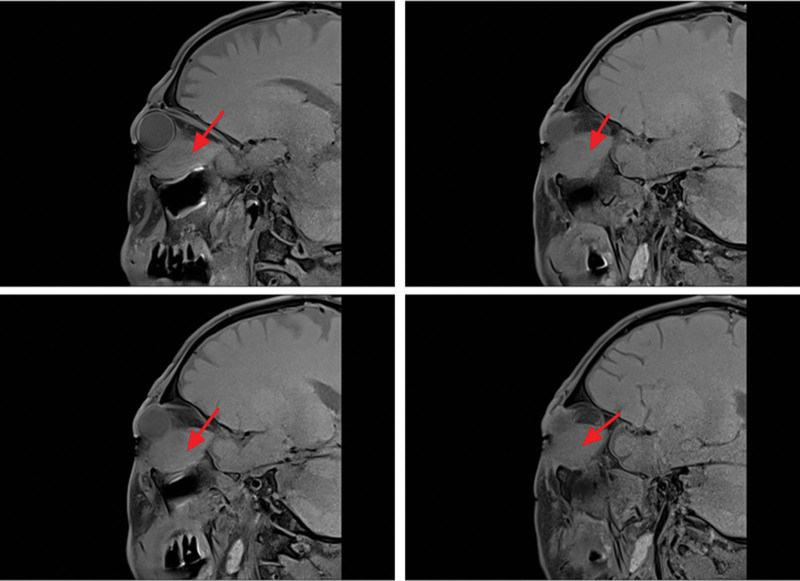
Patient MRI sagittal T1 phase with isosignal (red arrows) in the left posterior fat space, left lateral eye, and left inferior muscle space mass. MRI = magnetic resonance imaging.
